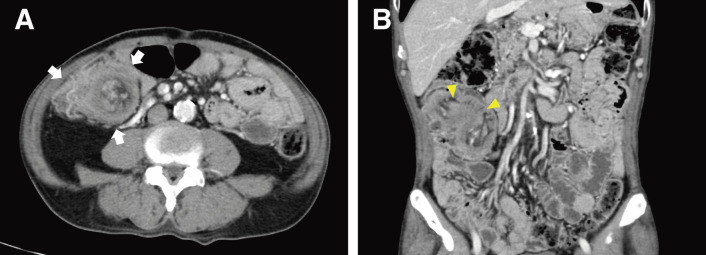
Contrast-enhanced CT scans. (A) The axial CT scan demonstrated a concentric, multilayered structure in the right colon (arrows), displaying the characteristic “target sign.” (B) The coronal CT image revealed an irregular mass within the hepatic flexure of the colon (arrowheads), accompanied by structures suggestive of mesenteric fat and vessels. CT, computed tomography
